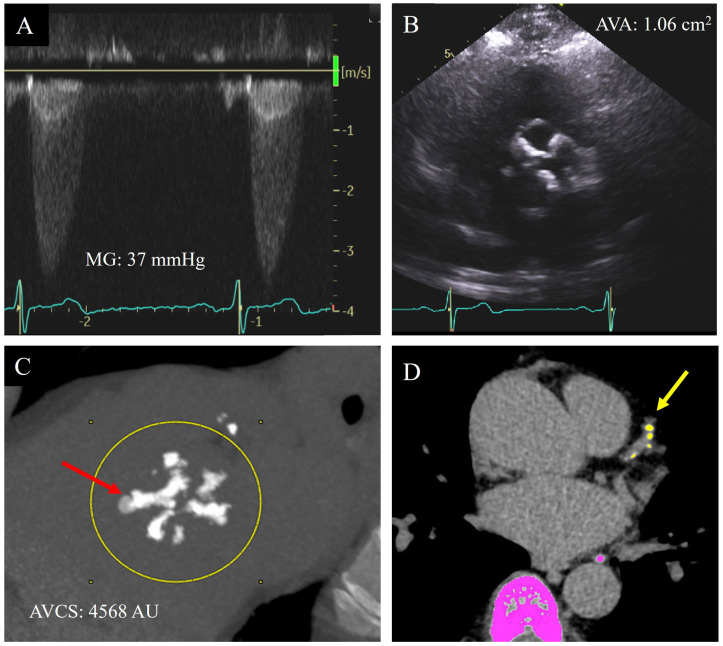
A 69-year-old male with bicuspid aortic valve and shortness of breath on exertion only at high elevations. He had discordant echocardiographic parameters for severity of aortic stenosis, with a clinical echocardiogram report noting overall moderate–severe aortic valve stenosis: systolic mean Doppler gradient (MG) 37 mmHg (A), aortic valve area (AVA) by Doppler 1.06 cm^2^ (B), dimensionless index 0.23, and normal indexed stroke volume (58 mL/m^2^). He proceeded to have an aortic valve calcium score (AVCS) by cardiac computed tomography ((C), red arrow) which demonstrated a score of 4568 AU, reclassifying aortic valve stenosis as severe. This scan also demonstrated calcification in the left anterior descending coronary artery ((D), yellow arrow).
